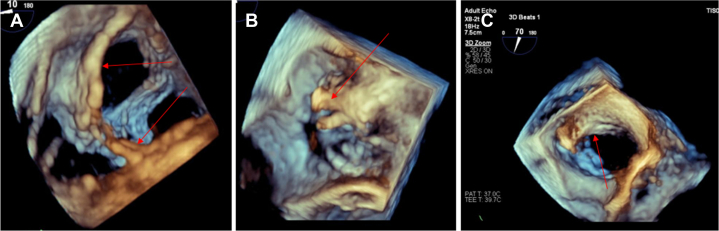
Transesophageal Echocardiography With 3D Reconstruction Focused on the Tricuspid Valve (A) Right ventricular view with insertion of the fibrous tissue (“ghost material”) on the tricuspid valve (red arrows). (B and C) Right atrial view with insertion of the fibrous tissue (“ghost material”) on the tricuspid valve and the atrial wall (red arrow).
